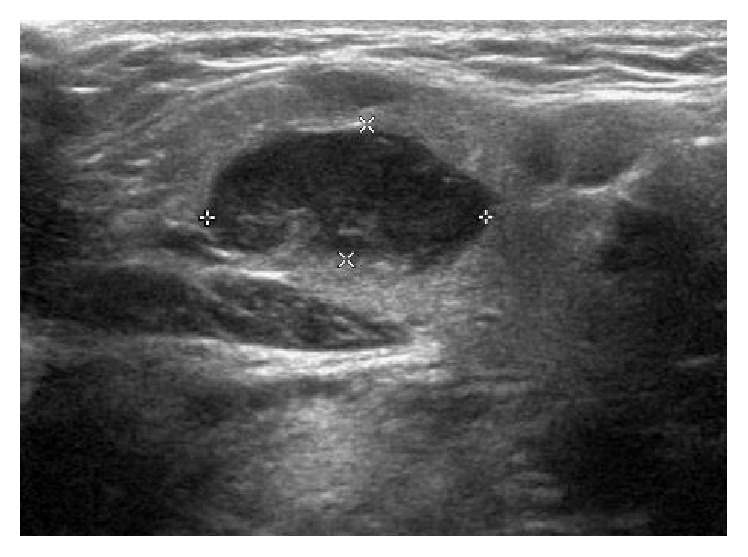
Diagnostic ultrasound of the left submandibular gland and neck.
